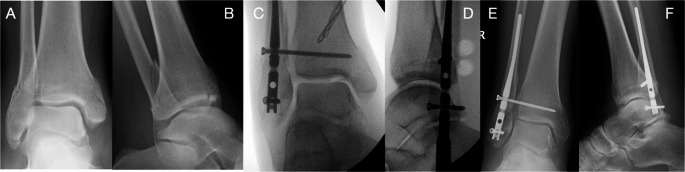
Preoperative anteroposterior (A) and lateral (B) radiographs and intraoperative radiographs (C and D) of a fibular nail fixation of an unstable ankle fracture. Weight-bearing anteroposterior (E) and lateral (F) radiographs taken at 6 months
